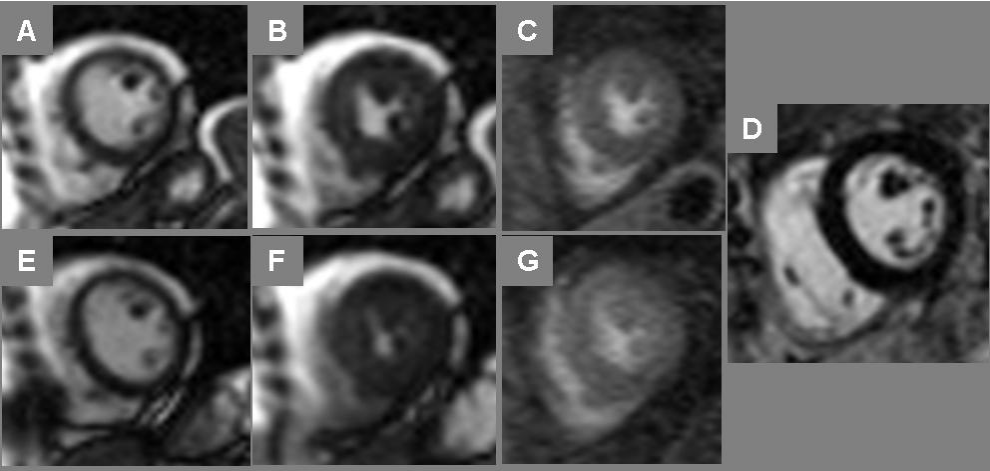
Normal Treadmill Stress CMR. End-diastolic (A, E) and end-systolic (B, F) frames of cine imaging at rest (top row) and immediately post-stress (bottom row) plus stress myocardial perfusion imaging (C, G) are shown in a 52 year-old postmenopausal female referred for stress SPECT to evaluate dyspnea; both stress modalities were negative for ischemia. In addition, late post-gadolinium enhancement (LGE) CMR imaging (D) showed no myocardial enhancement.
